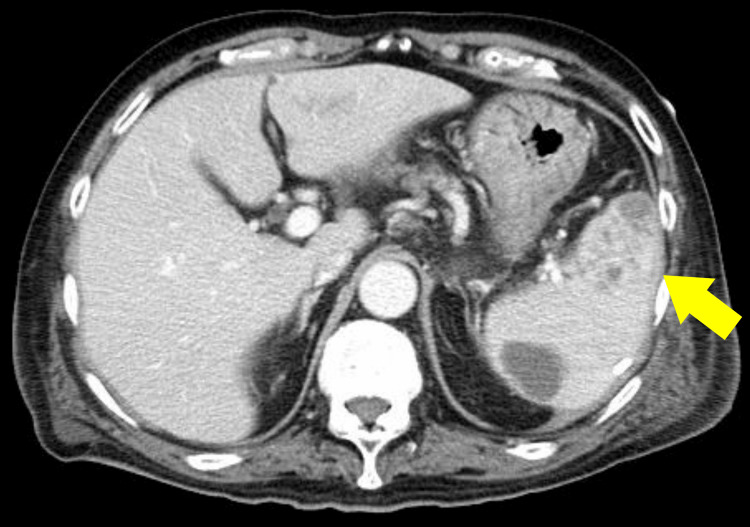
CT findings after percutaneous drainage on hospital day 9. The portal venous gas and free intraperitoneal air had disappeared, but the intrasplenic abscess had enlarged to approximately 6 cm in maximum diameter (yellow arrow).
